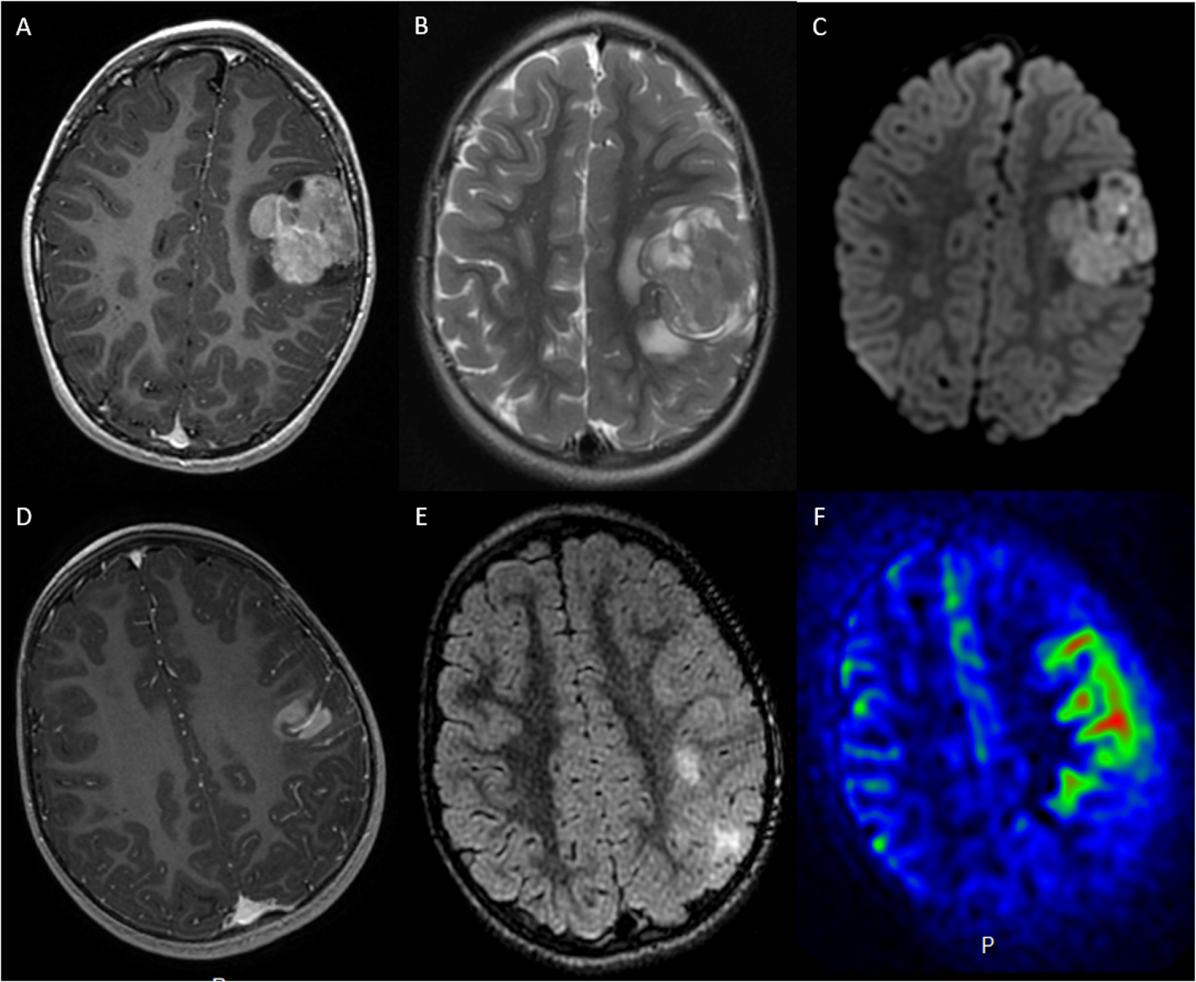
Radiological features of two supratentorial HGG-MYCN. First line: Case 3. (a) T1-weighted images after contrast media injection, (b) T2-weighted images, and (c) diffusion-weighted images: a solid lesion with peri-lesional edema, homogeneous enhancement and hypercellularity (apparent diffusion coefficient (ADC) on diffusion weighted images is restricted in the main part of the tumor). Second line: Case 1. (d) T1-weighted images after contrast media injection, (e) FLAIR-weighted images and (f) cerebral blood flow map using arterial spin labeling: a solid and infiltrative lesion with homogeneous enhancement and high cerebral blood flow
